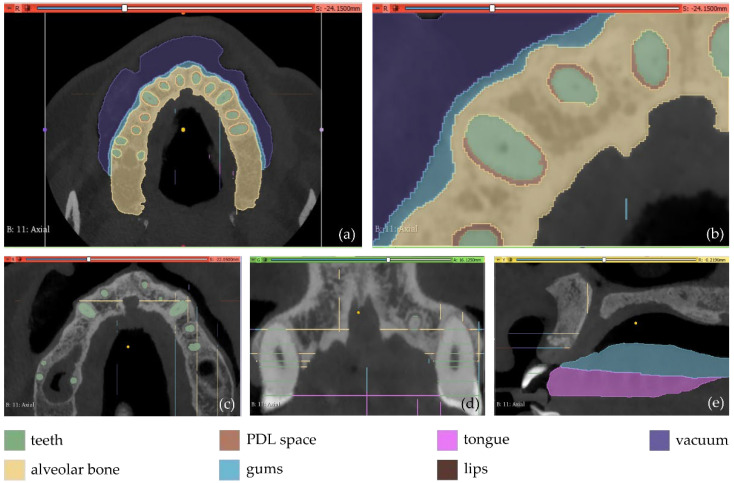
Manual definition of segments in 3D Slicer using CBCT slices of the maxilla of a patient within Experiment 1; (a): a CBCT slice in the axial plane; (b) zoom-in view of the CBCT slice in the axial plane, shown in (a); (c) zoom-in view of another CBCT slice in the axial plane; (d) zoom-in view of a CBCT slice in the coronal plane; (e) zoom-in view of a CBCT slice in the sagittal plane.
